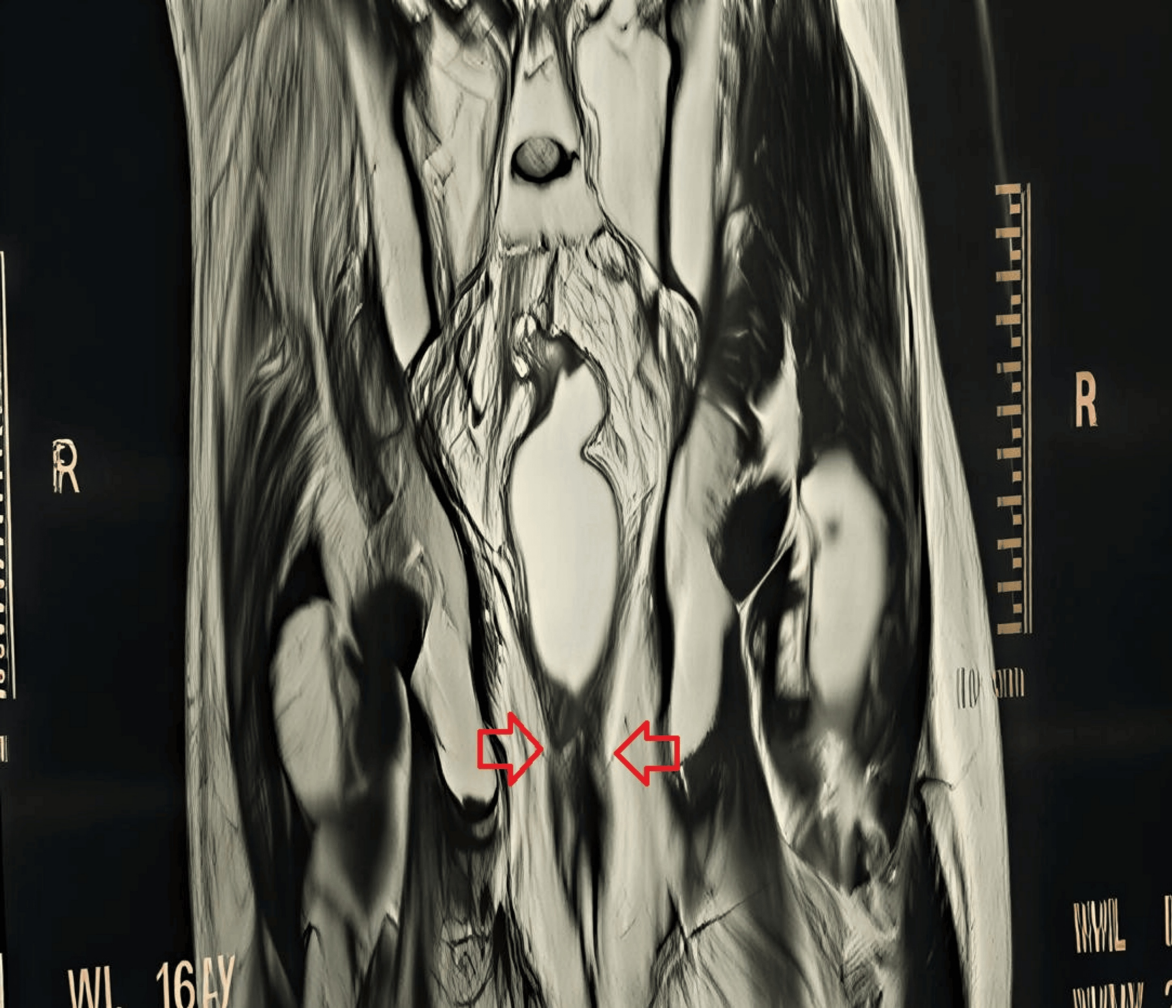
Sagittal section of pelvic magnetic resonance imaging (MRI) demonstrating a tumor of the distal rectum.
